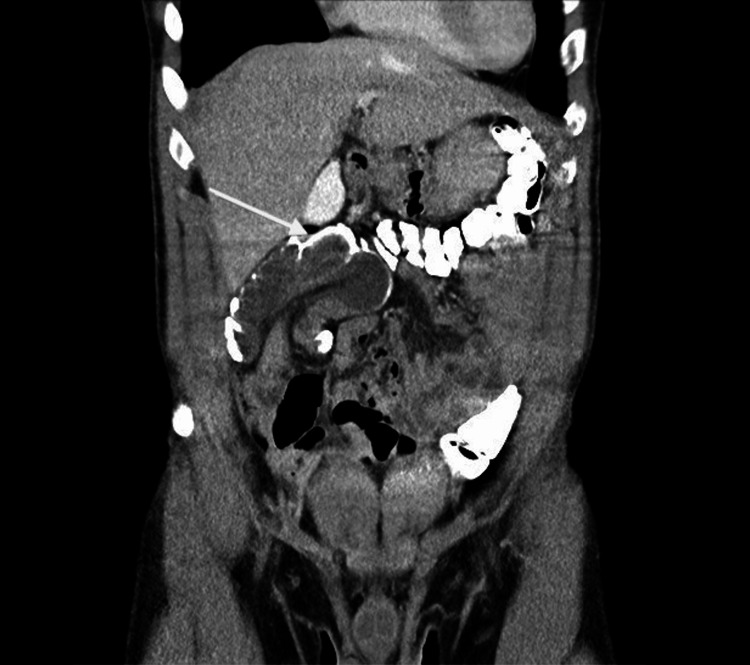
Coronal CT abdomen with IV contrast shows persistent large ileocolic intussusception.
